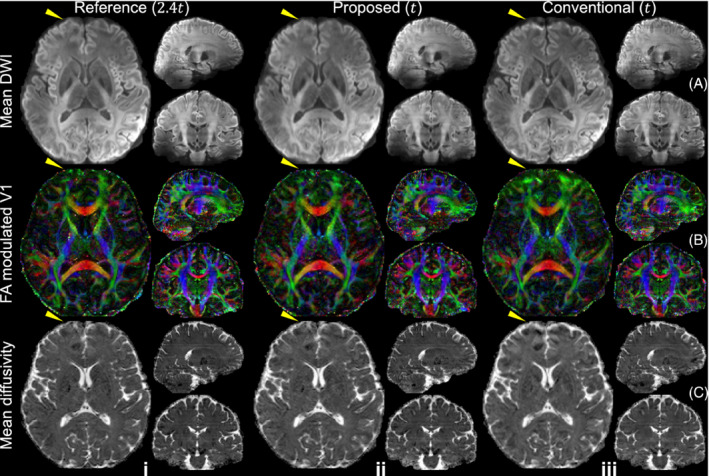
Comparisons of whole‐brain diffusion images. The mean diffusion‐weighted image (DWI) (A), the fractional anisotropy (FA) modulated primary eigenvector (V1) (B), and the mean diffusivity (C) of diffusion data acquired using blip‐up and blip‐down k_z_‐fully sampled (i.e., 24 blip‐up and 24 blip‐down shots for each diffusion direction) reference protocol (Reference, i), joint blip‐reversed with CAIPI‐PF protocol (Proposed, ii) and conventional 3D multi‐slab protocol using only blip‐up phase encoding (Conventional, iii) at 1.22 mm isotropic resolution from the same subject are displayed. The parameter *t* represents the scan time of a rectangular sampling without over‐sampling or acceleration along k_z_ for one phase encoding direction (as Figure 2C,i). The yellow arrows highlight the regions with strong distortions near frontal which cannot be fully corrected with the conventional method.
